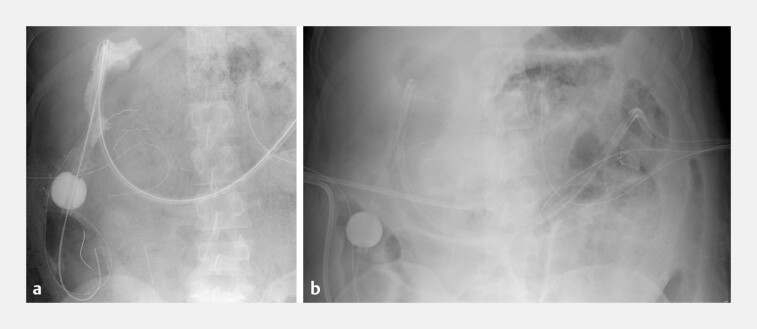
Fluoroscopic and radiographic images of ileus tube placement for fecal diversion.
